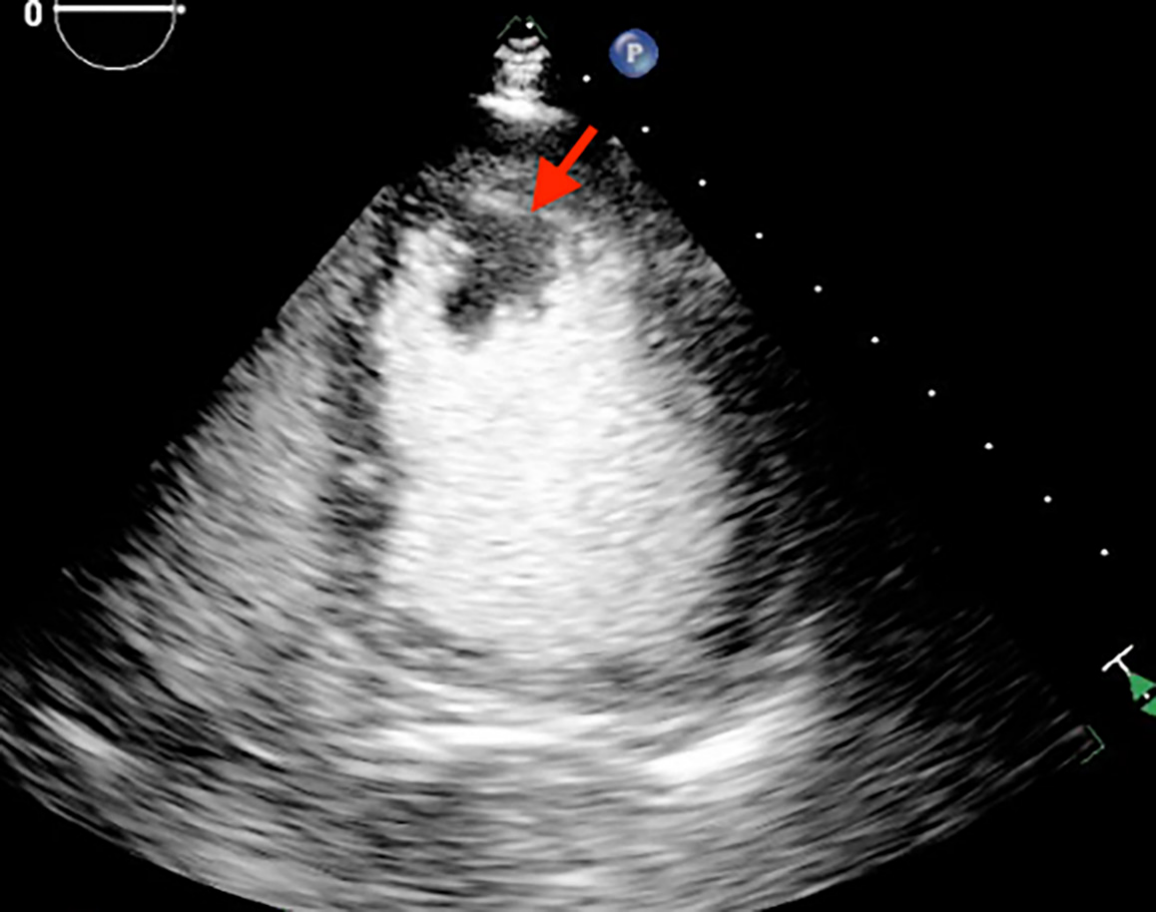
Transthoracic echocardiogram two chamber view with contrast demonstrating an irregular distal left ventricular mass (arrow).
